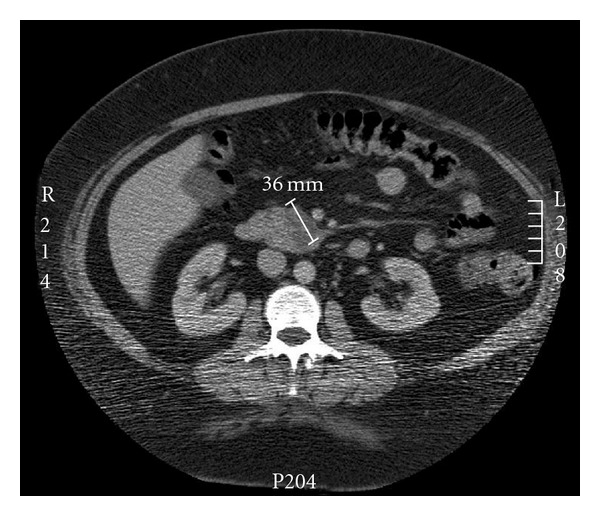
CT scan of the abdomen showing a 3.6 × 2.4 cm cystic lesion within the uncinate process of the pancreas extending into the pancreatic head.
